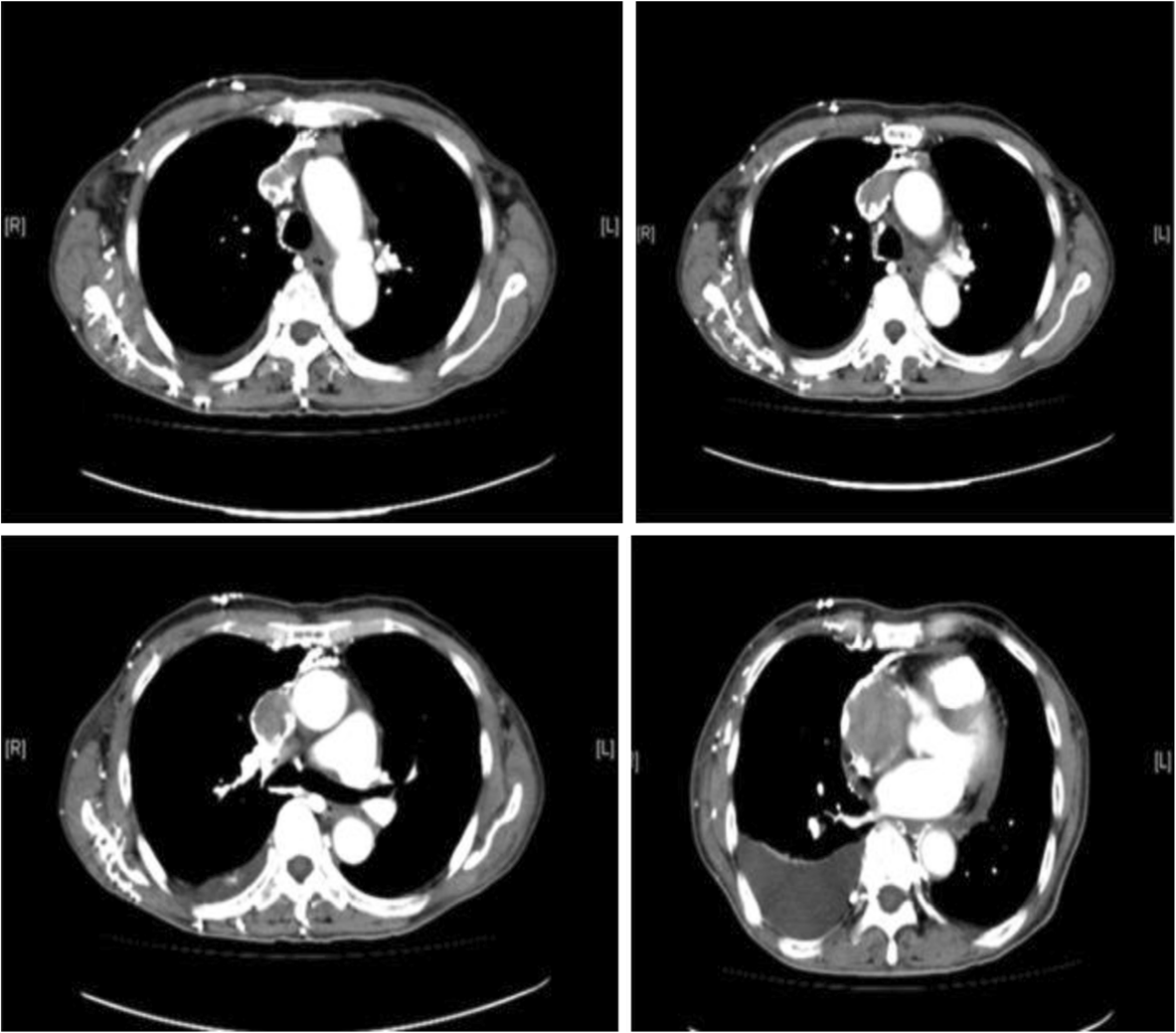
Computed tomographicscan revealed a large, well-defined, round softtissue density mass in the left brachiocephalic vein, superior vena cava, and right atrium.
